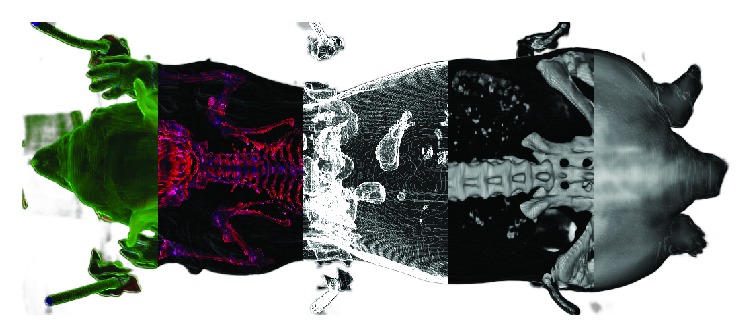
Selection of different renderings of CT data produced by the included volume renderer node and some supporting nodes. From left to right: solid with curvature as color; solid rescaled with curvature as color; edge/step function; solid rescaled; translucent.
